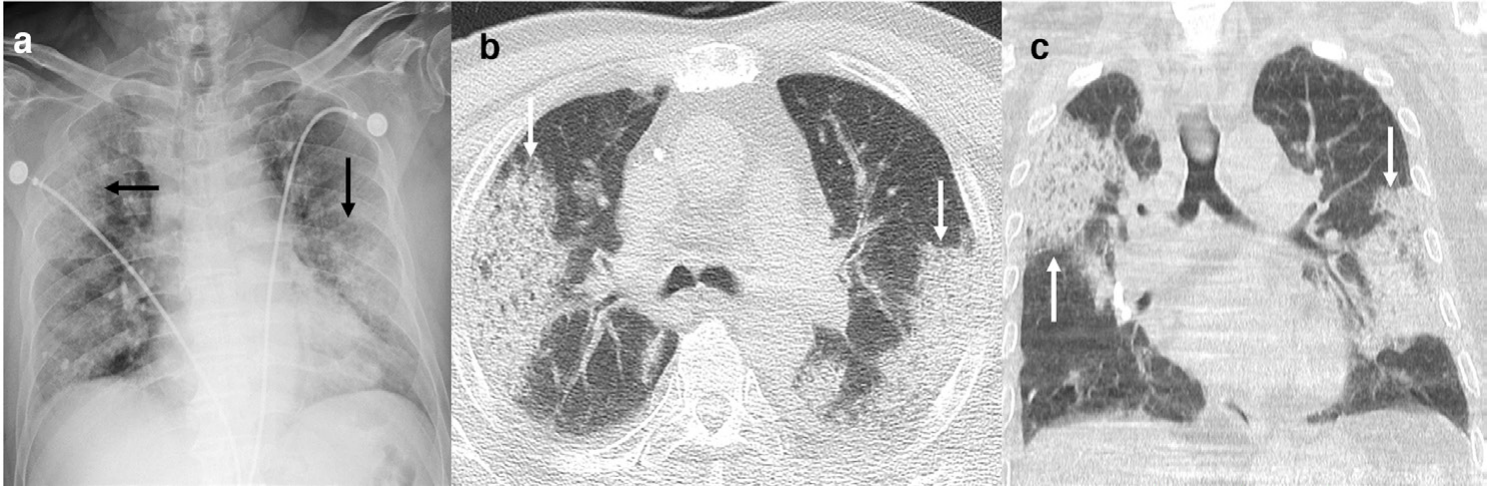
Chest X-ray obtained two weeks after diagnosis of COVID-19 infection showing dense bilateral opacification in peripheral lungs (black arrows). Axial (B) and Coronal (C) CT chest images (from same day) showing bilateral peripheral consolidation (white arrows) without pleural effusion or lymphadenopathy.
